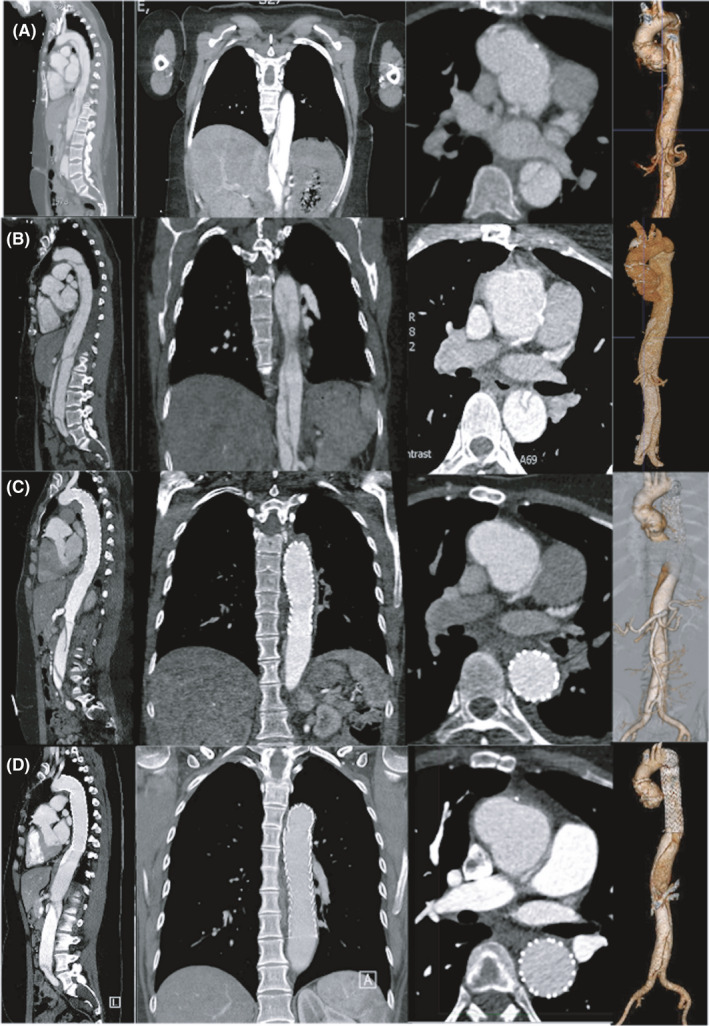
Diagnostic contrast computed tomography (CT) shows acute type B aortic dissection with a flap originating at the origin of the left subclavian artery and extending beyond the aortic bifurcation into both common iliac arteries (panel A); follow‐up CT at day 7 reveals further dilatation of the dissected descending thoracic aorta with compression of the true lumen at mid‐descending thoracic aorta level (panel B); Post‐TEVAR CT demonstrates complete exclusion of the false lumen without malperfusion (panel C); follow‐up CT at 7 months shows an excellent result and aortic remodelling with no distal expansion of the abdominal remainder of the dissection (panel D)
